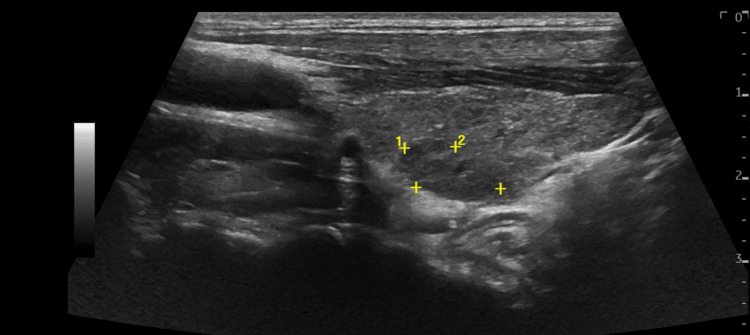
Left thyroid nodule A 1.3 x 0.8 cm hypoechoic homogenous nodule with well-defined borders was identified on ultrasound examination of the neck.
